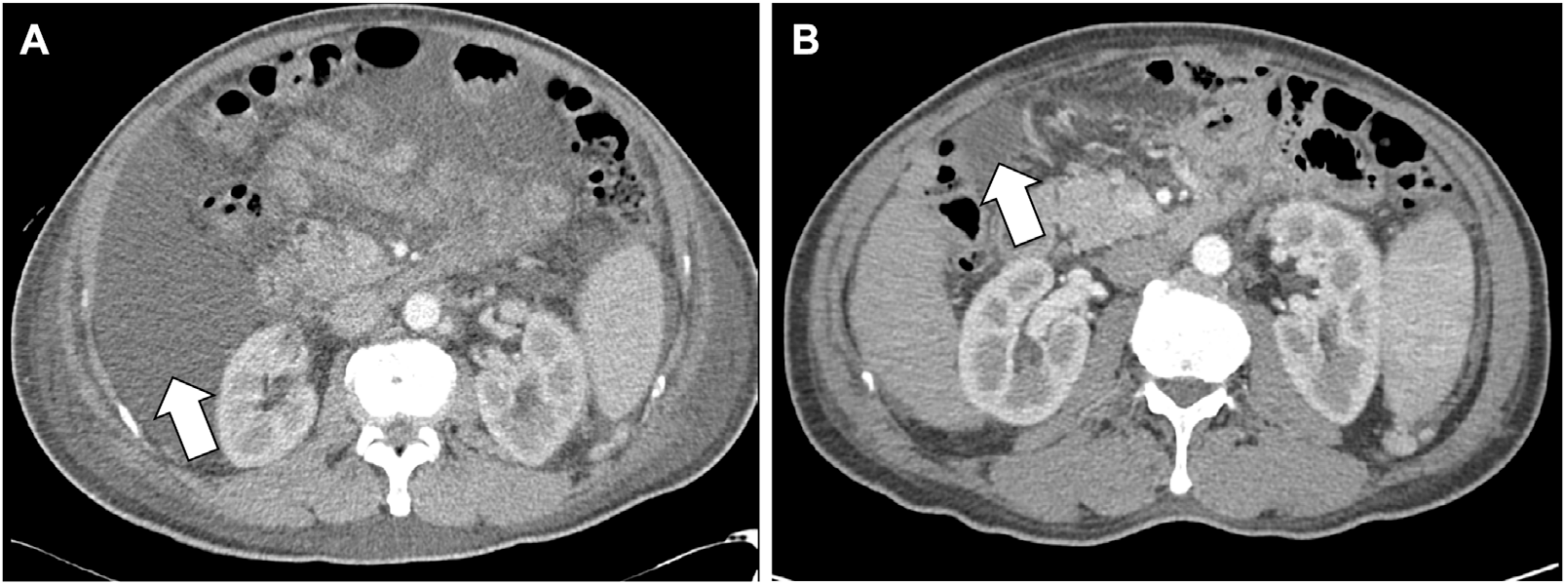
Decrease of ascites in the CT scan images. (A) Is an abdomen CT image which were scanned on March 8 (B) is a follow-up abdomen CT image which were scanned on July 23. White arrows indicate a shrinkage of ascites in (B), compared to (A). Abbreviations: CT, computed tomography.
